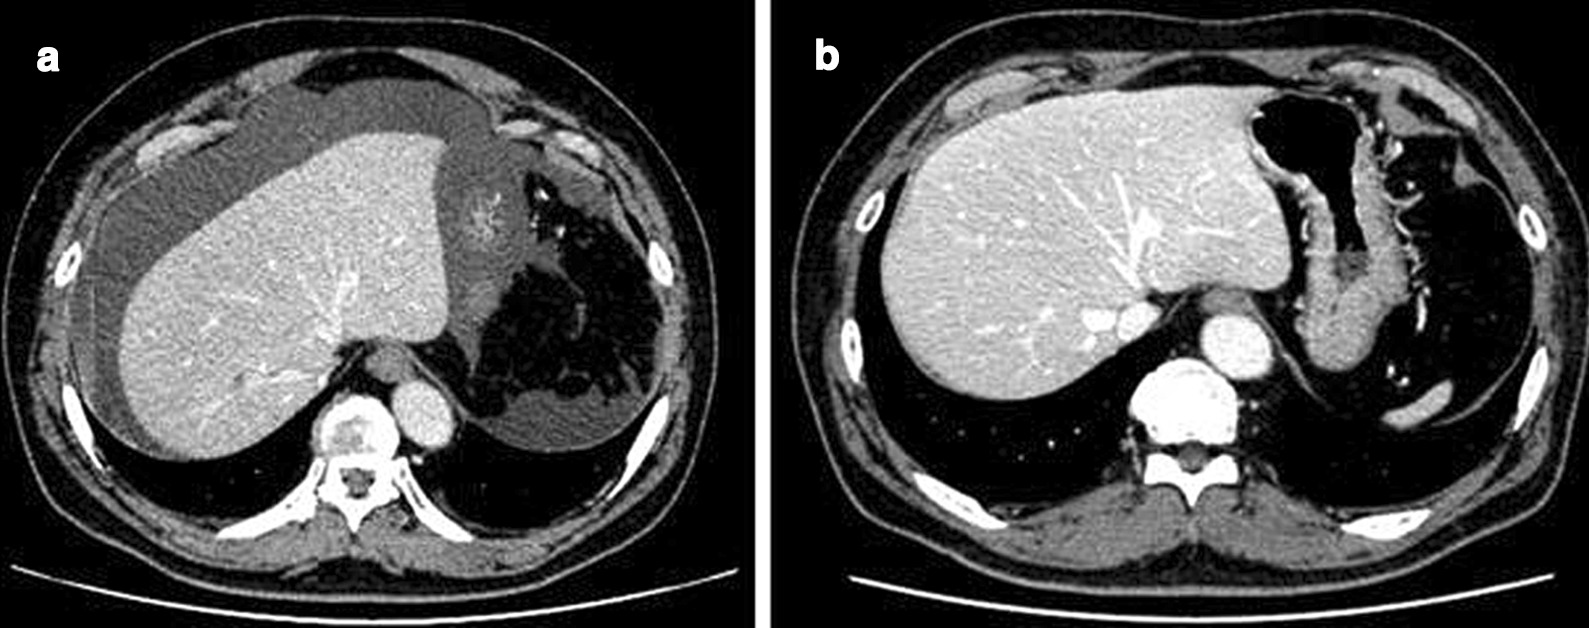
Abdominal CT with contrast. (a) Before steroid administration. (b) One and a half months after steroid administration
